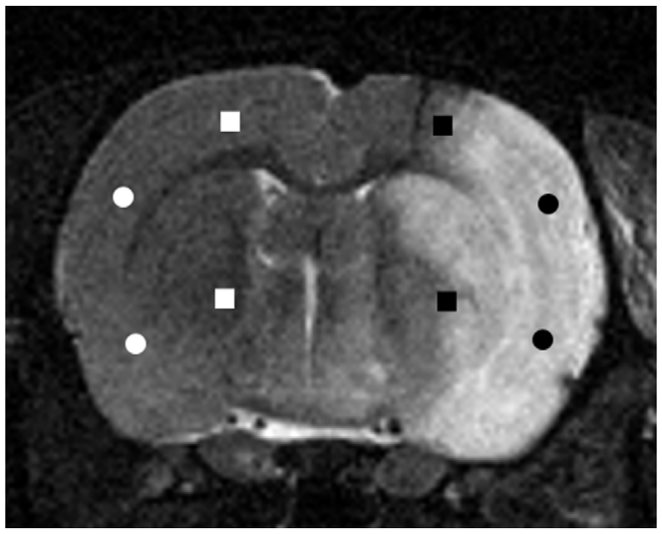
Representative T2-weighted, axial magnetic resonance image 24 h after middle cerebral artery occlusion with reperfusion. The hyperintensity corresponds to the ischemic area. Tissue was obtained from the ischemic core (black circles), the border of the ischemic lesion (black squares) and areas corresponding to the ischemic core (white circles) and its border (white squares) in the contralateral, non-ischemic hemisphere.
